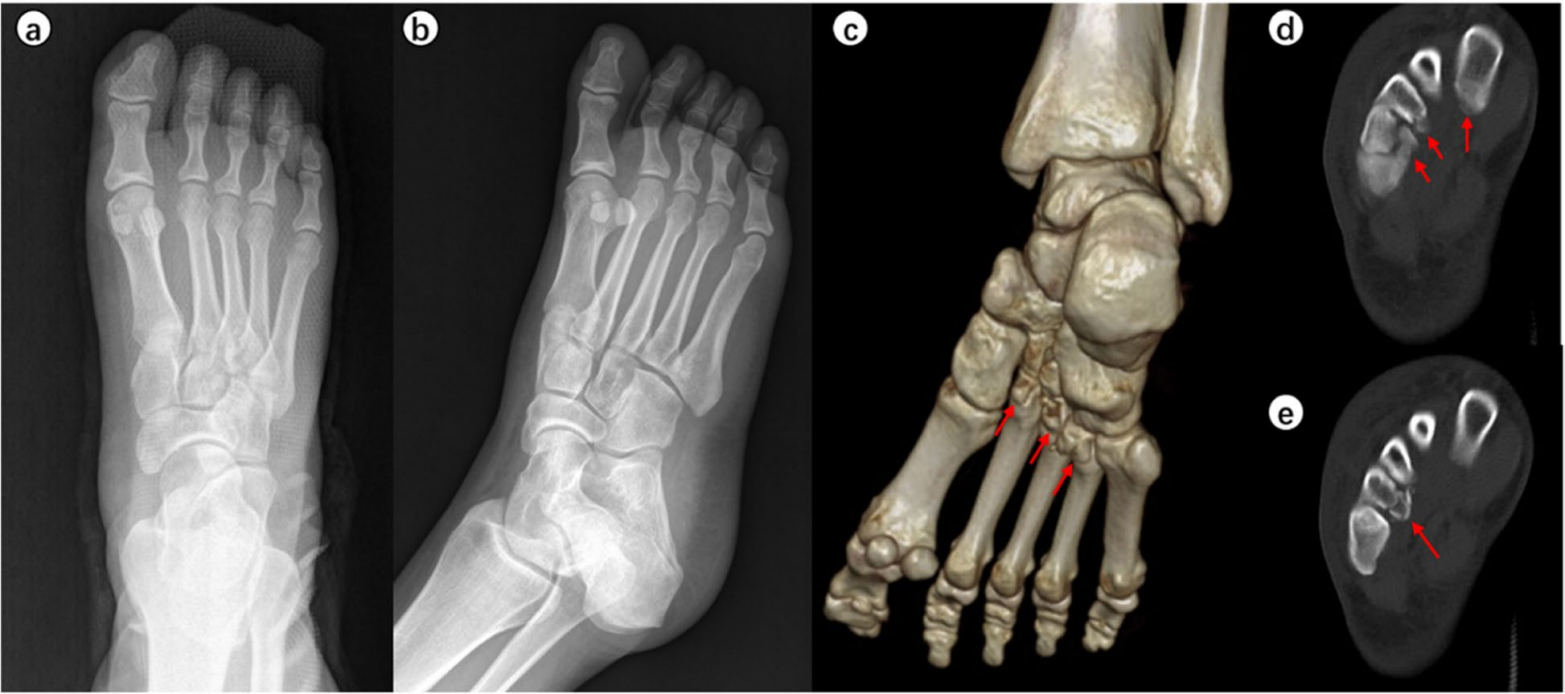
A 30-year-old female hurt her right foot after slipping. Chiodo-Myerson’s classification: three-column injury; Displacement classification: non-displaced injury. Observer A made the wrong diagnosis for two times, and changed the initial treatment option (conservative treatment to surgery) after evaluating CT image. Observer B made the correct diagnosis at the second time, and changed the initial treatment option (conservative treatment to surgery) after evaluating CT image. a-b The Lisfranc injury was easily missed on plain X-ray. c-e The plantar intra-articular fractures of the base of the first to fourth metatarsal bones are shown on computed tomography images. The red arrows indicate the fracture fragments
